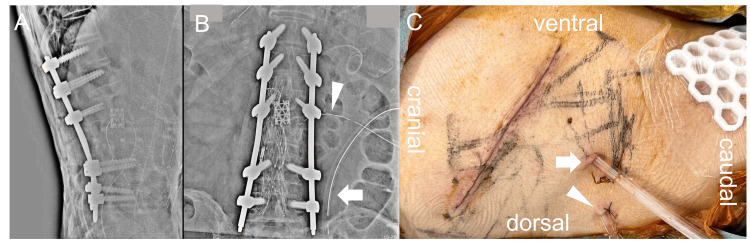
Postoperative lumbar spine radiographs and a photograph of the surgical site (A) lateral view, (B) anterior-posterior view; postoperative lumbar spine radiographs. (C) postoperative photograph of the surgical site. Arrow: 24 Fr dual-lumen tube going to the iliopsoas abscess; arrowhead: 16 Fr dual-lumen tube going to the intervertebral disc.
